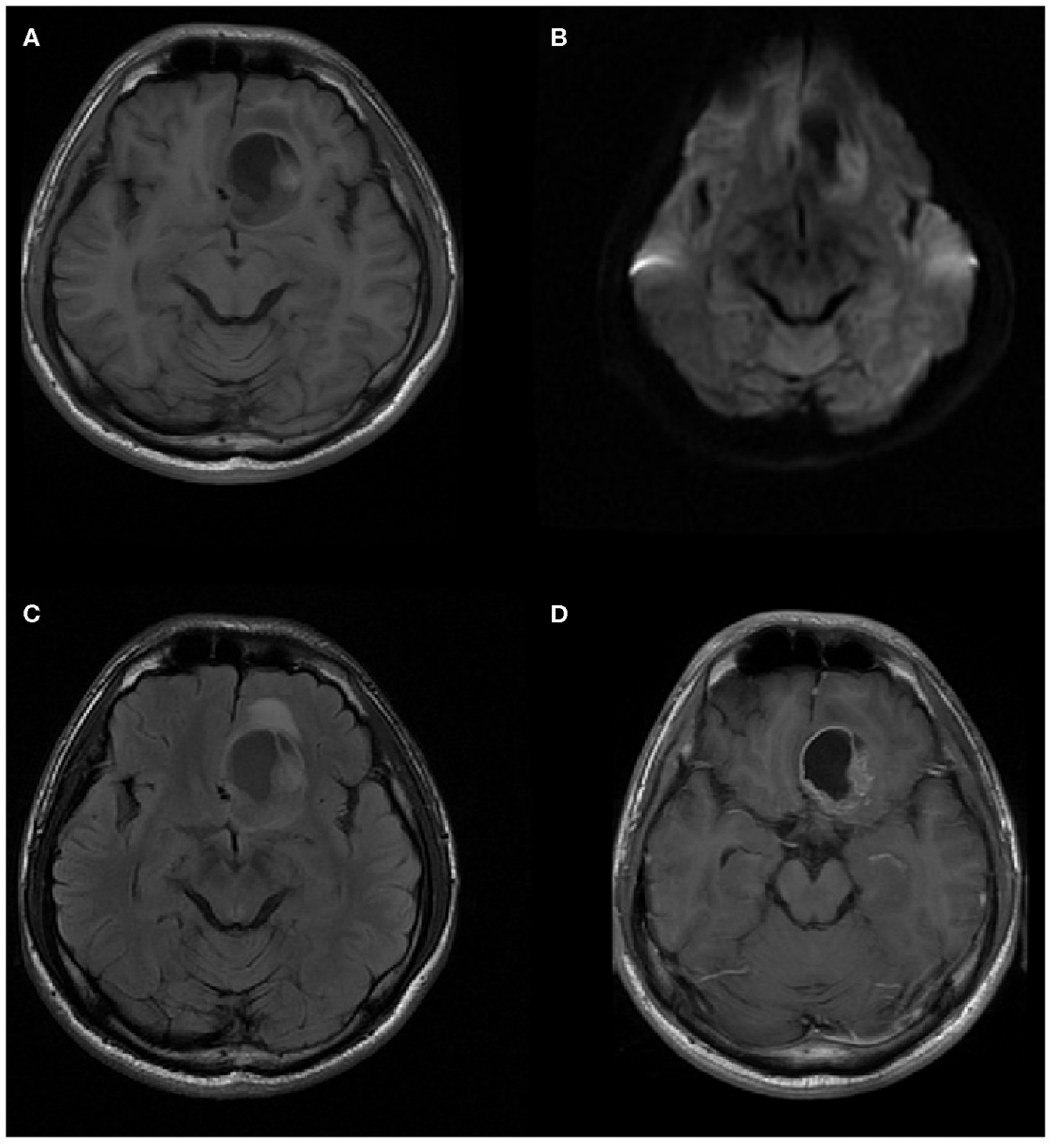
A 20-year-old man with supratentorial intraparenchymal anaplastic ependymoma in the left frontal lobe on (A) T1-weighted image, (B) diffusion-weighted images, (C) fluid-attenuated inversion recovery (FLAIR), and (D) contrast T1-weighted image. The tumor appears as heterogeneous signal intensity, big cysts, reduced diffusion within the soft tissue component, and mild peritumoral edema. There is an area of focal hyperintensity within the tumor, representing hemorrhage.
